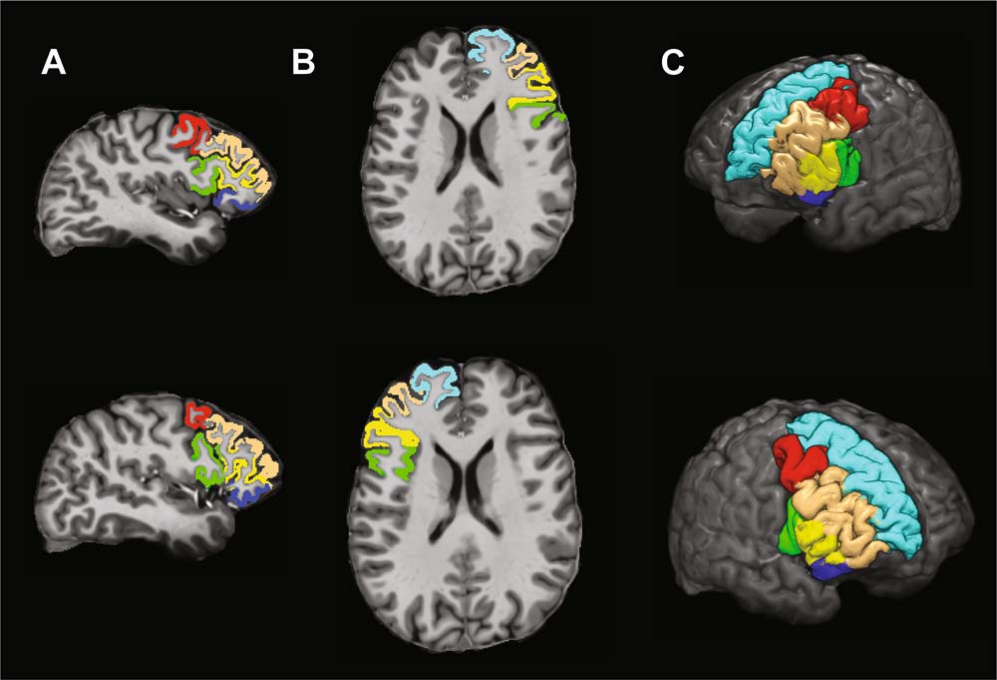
Regions of interest (ROIs) for cortical thickness analysis. A) Sagittal and B) axial views of the brain depicting the ROIs in both hemispheres. C) 3D lateral view of the brain showing all ROIs. Colors represent caudal middle frontal (red), pars opercularis (green), pars triangularis (yellow), rostral middle frontal (orange), superior frontal (light blue), and pars orbitalis (dark blue). Top row shows the left hemisphere; bottom row shows the right hemisphere. Cortical thickness measures were extracted from these ROIs using advanced normalization tools (ANTs) and the DKT-31 atlas. Median cortical thickness values across three MRI sessions were used for each participant.
